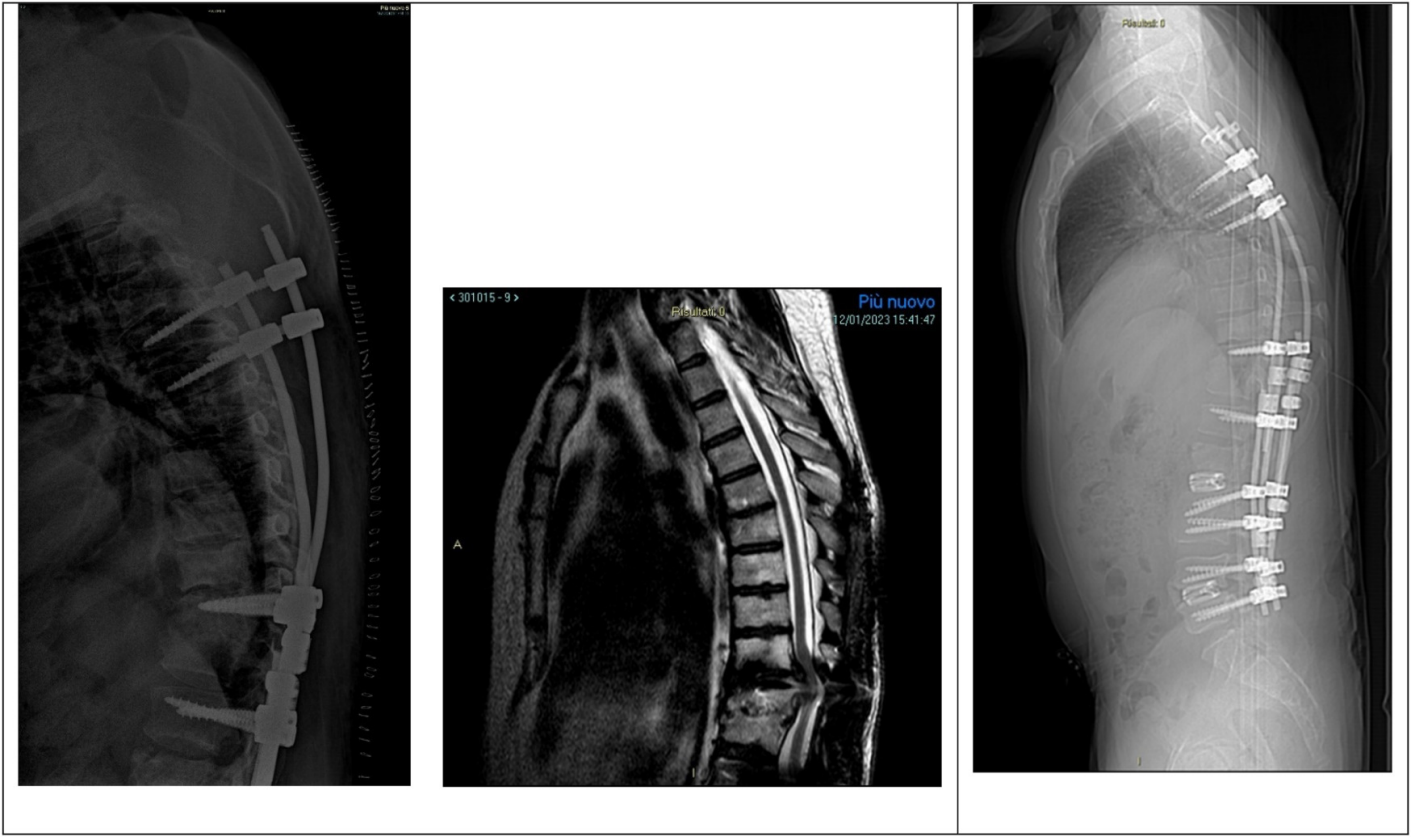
Left, mobilization of the proximal instrumentations with screws loosening on the left side. Center, MRI showing PJK with T9–T10 spinal cord compression. Right, postoperative x-ray showing proximal extension of the instrumentation to T4 and decompression T9–T10.
